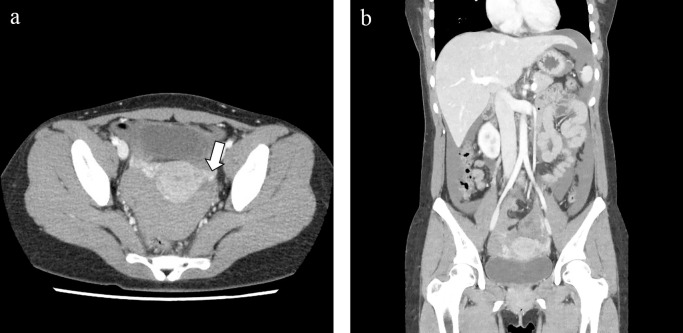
Abdominal contrast-enhanced CT scan: a) Findings suggestive of contrast extravasation (⇨) were observed around the left adnexa. b) A large volume of ascites was observed extending around the liver and spleen.
